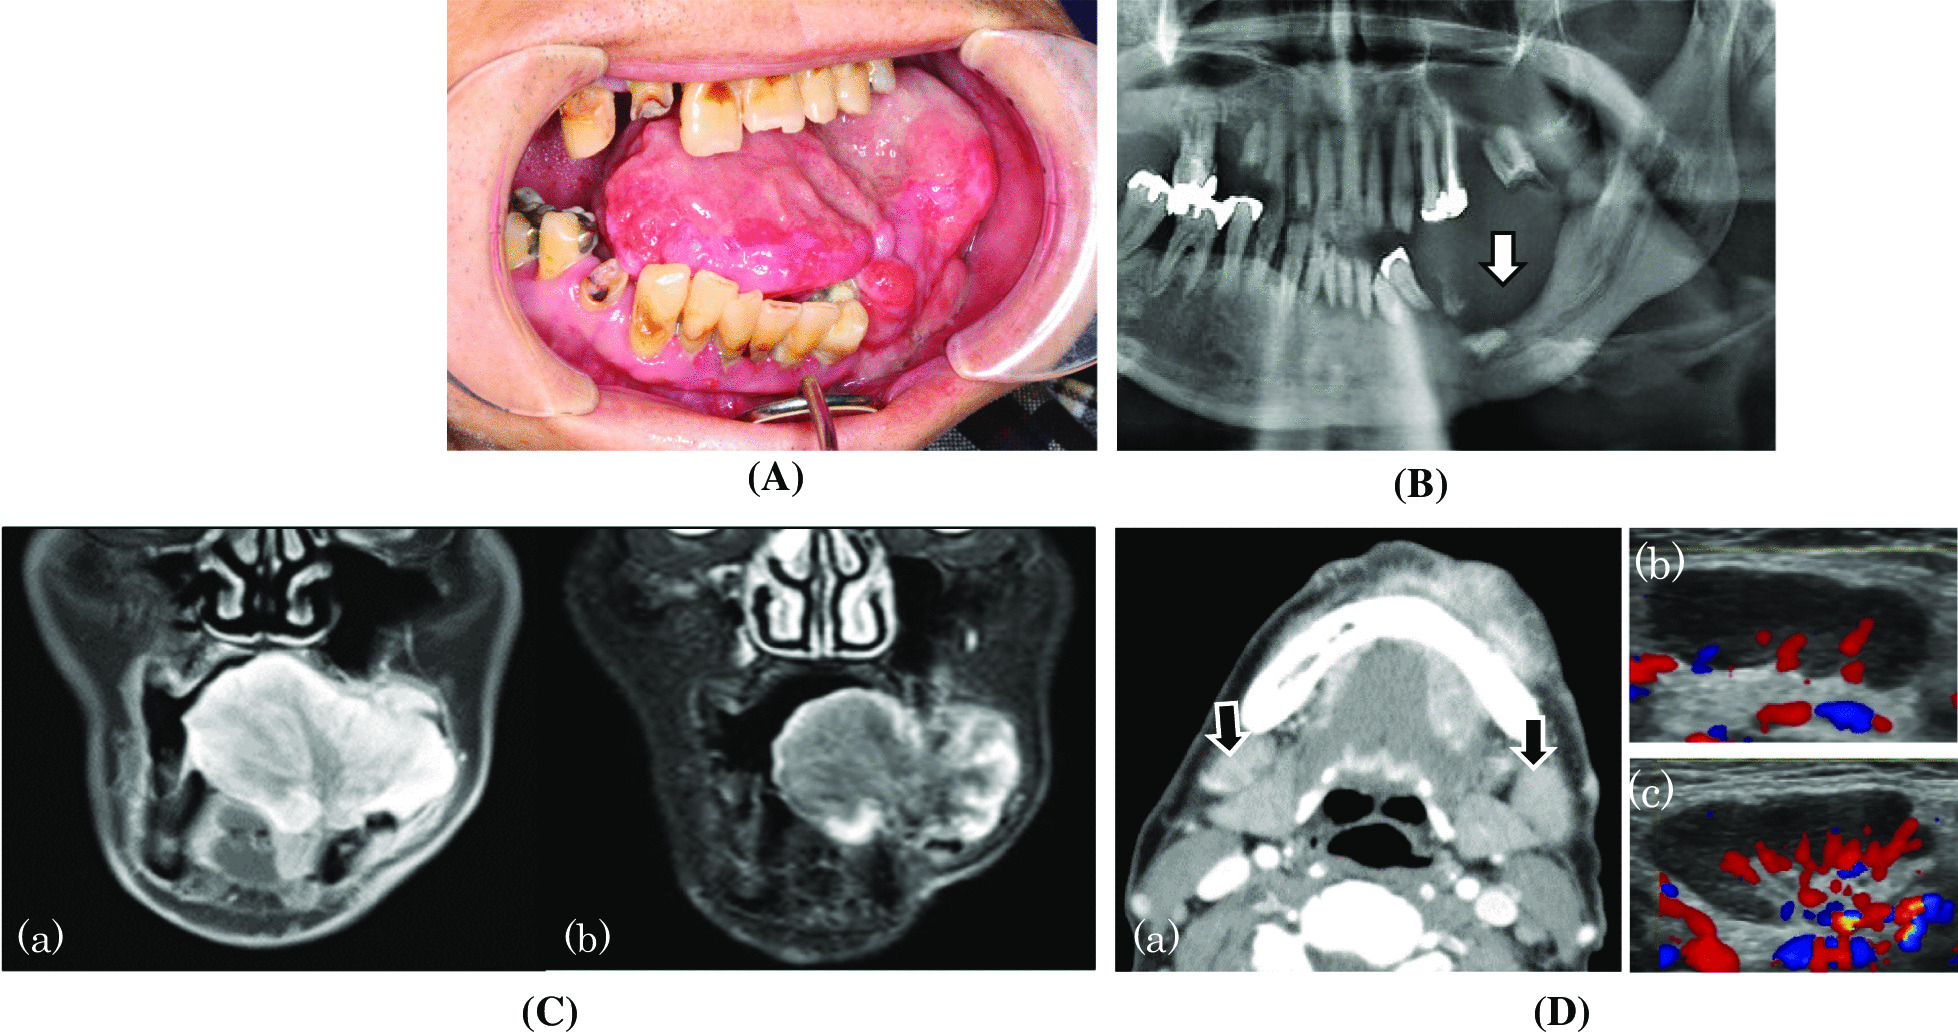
Patient status. A Oral findings at first examination: a pedunculated tumour (approximately 65 × 45 mm) is present in the left lower gingiva. The base is localised in the alveolar region, and the surface is granulomatous, elastic-hard with partial ulcerations, and haemorrhagic. The tongue is displaced rightward by the tumour (arrow). B Panoramic radiography revealed compressive bone resorption in the region corresponding to the tumour (arrow). C MRI images: (a) Contrast T1-Gd-MRI revealed significant contrast throughout the tumour, more pronounced along the margin; (b) T2-STIR-MRI revealed a high intensity at the margin and moderate intensity in the inner region. D Imaging of the submandibular lymph node: (a) in the contrast-enhanced CT image, contrast-enhanced swollen lymph nodes (arrows) are present in the bilateral submandibular regions. Both the right (b) and left (c) submandibular lymph nodes are soft, with smooth margins on cervical echo. The hilum of the lymph node is clear, but an increase in blood flow is noted in the hilum region. T1-Gd-MRI: T1-weighted gadolinium-enhanced magnetic resonance imaging, T2-STIR: T2-weighted short inversion recovery
